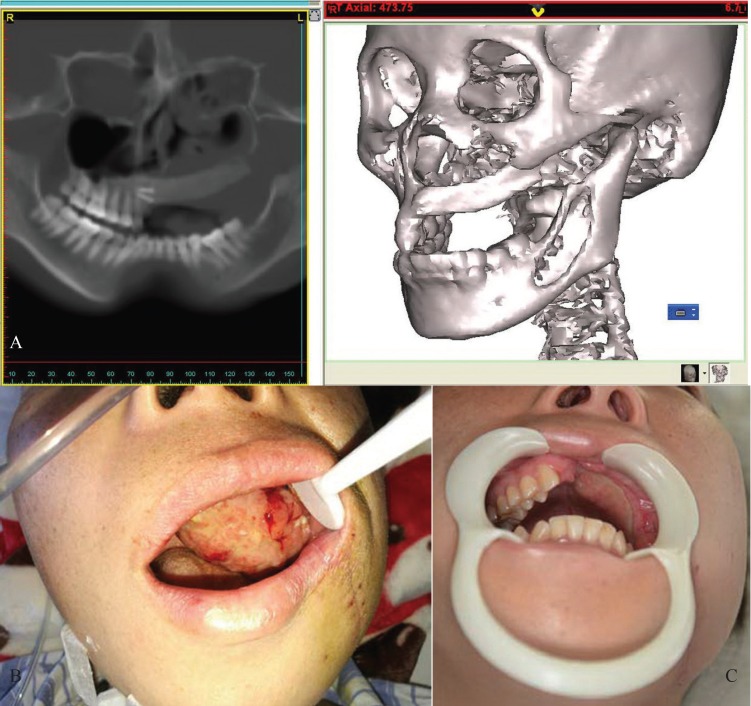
A. Three-dimensional computed tomography scan confirmed volume maintenance and anatomic continuity of the graft. B. The frontal view two weeks later. C. Six months later.
